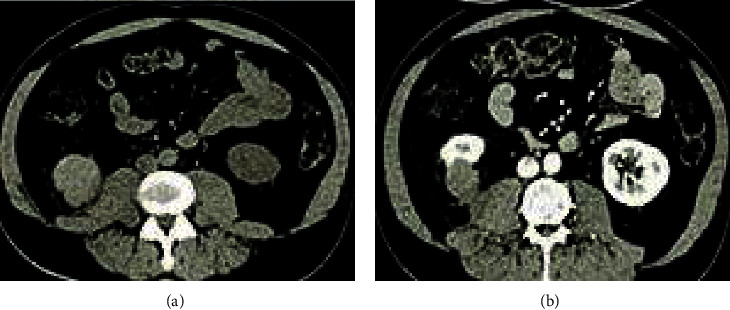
CT image of a patient's kidney (male, 51 years old). The left image was the CT plain scan result, and the right image was the CT enhanced result.
